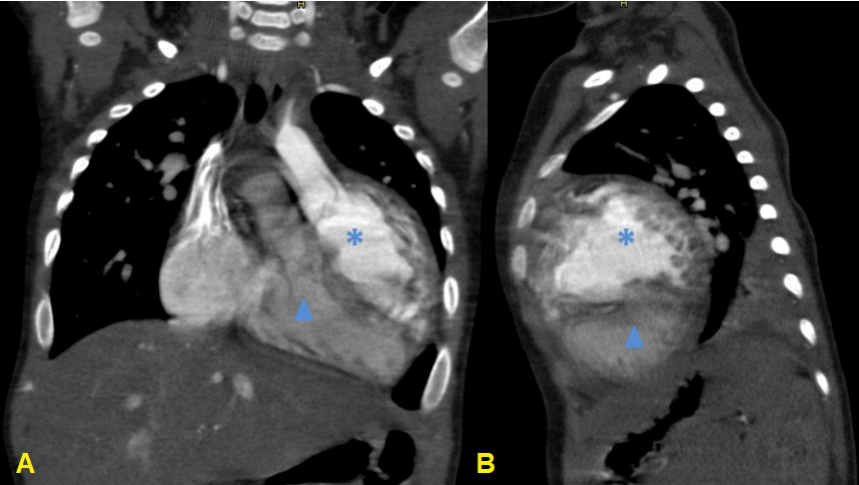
Computed tomography angiography: coronal (A) and sagittal (B)
